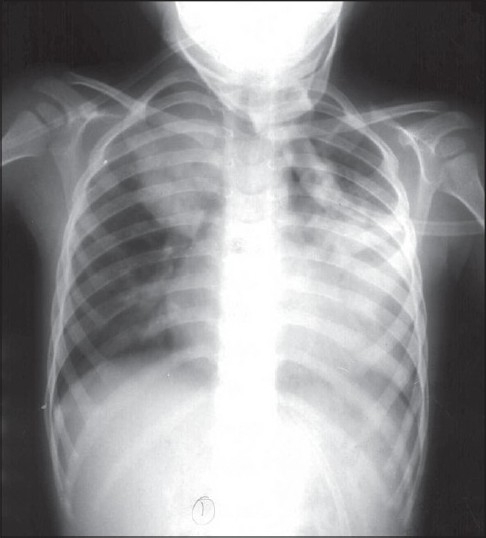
Chest radiograph on admission showing right upper and middle zone pneumonia along with pleural effusion on left side.
